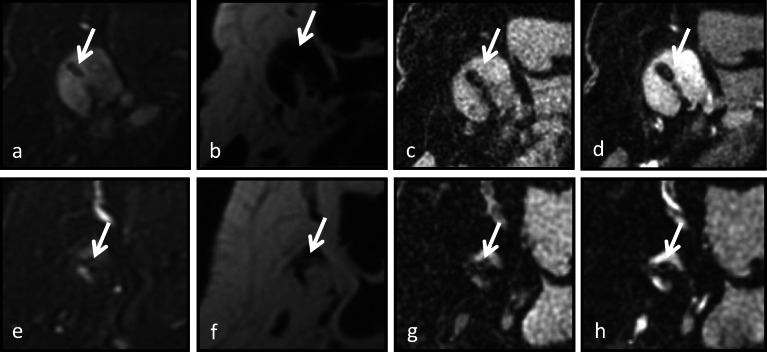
A 49-year-old female with invasive lobular carcinoma of the right breast with biopsy proven right axillary metastatic lymphadenopathy. A Tumark x-shaped marker was placed at site of axillary lymph node biopsy. a–d Initial staging magnetic resonance imaging (MRI) 2 weeks after lymph node marker placement. The marker is seen with high confidence on the T2-weighted water-only fast spin-echo image (a), the unenhanced fat-suppressed three-dimensional T1-weighted spoiled gradient-recalled image (c), and the first contrast-enhanced fat-suppressed three-dimensional T1-weighted spoiled gradient-recalled image (d), while it is seen with low confidence on the T2-weigheted fat-only fast spin-echo image (b). In the follow-up of neoadjuvant therapy, MRI was performed 6 months after biopsy marker placement. The marker is seen with low confidence on all images: T2-weighted water-only fast spin-echo image (e), T2-weighted fat-only fast spin-echo image (f), unenhanced fat-suppressed three-dimensional T1-weighted spoiled gradient-recalled image (g), and first contrast-enhanced fat-suppressed three-dimensional T1-weighted spoiled gradient-recalled image (h), with poor visualization attributed to the decreased size of lymph node cortical thickness
